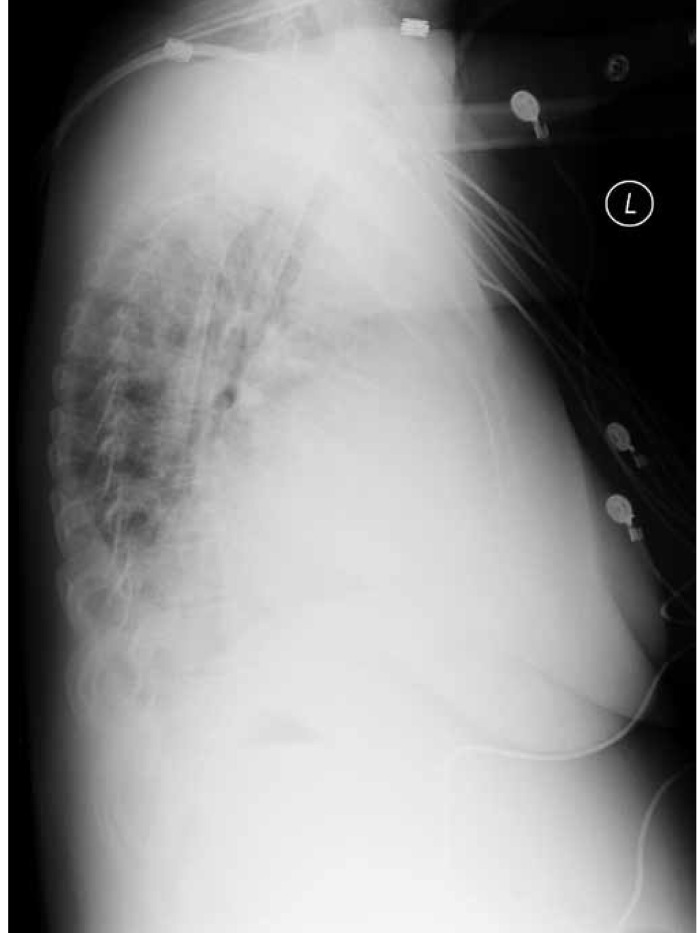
Chest X-ray lateral view.
